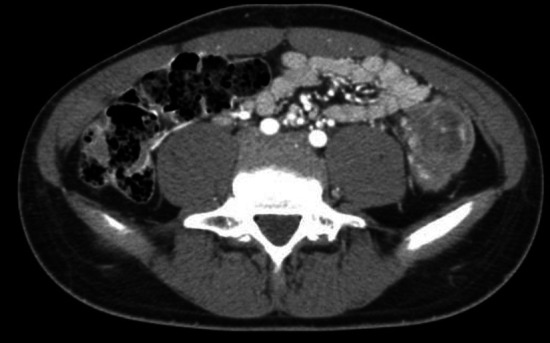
Abdominal CT-scan image demonstrating neoplastic mass in the descending colon which measures 5 × 3 cm
